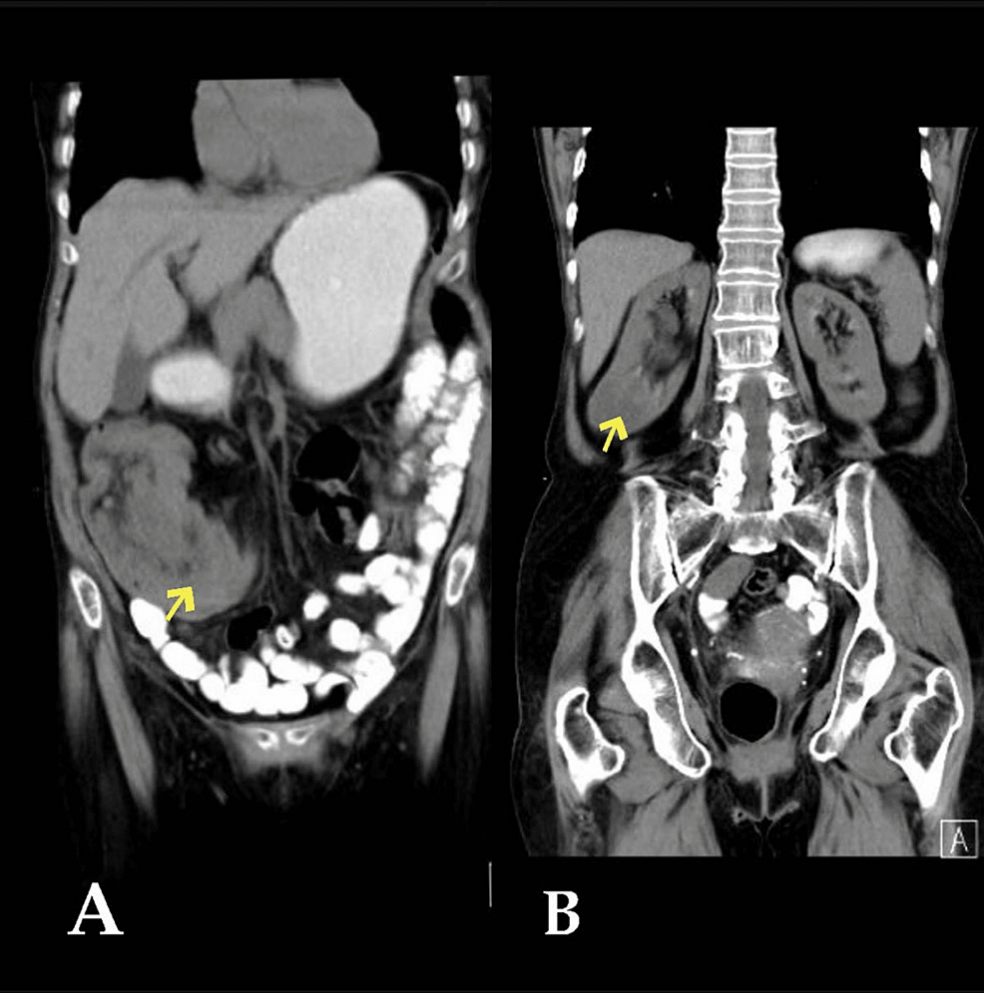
Case 2 - pre- and post-contrast CT scan of the abdomen. The images show (A) ileocecal intussusception reaching the mid-transverse colon with a suspected lesion at the apex of the invaginate bowel and (B) a rounded exophytic mass lesion from the lower pole of the right kidney.
